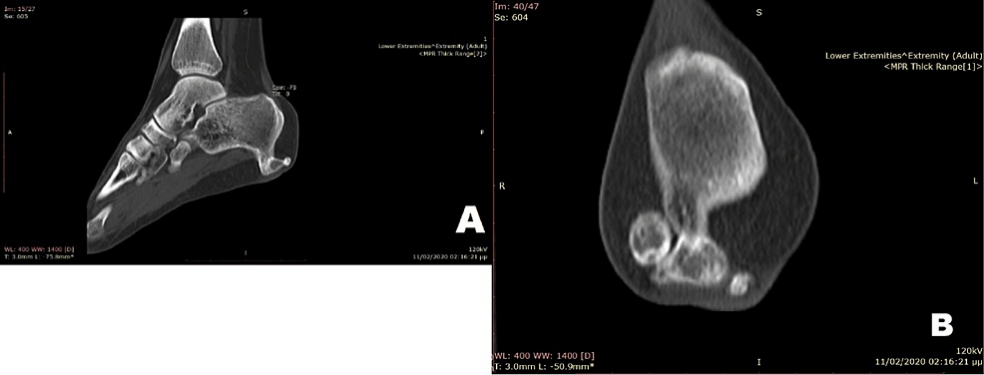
A preoperative CT scan of the affected foot A preoperative sagittal (Figure 2A) and coronal (Figure 2B) CT scan of the foot without contrast material Images demonstrate a pedunculated osteochondroma with no visible cartilage cap. Measured dimensions: anteroposterior 45 mm, superoinferior 25 mm, and mediolateral 30 mm
